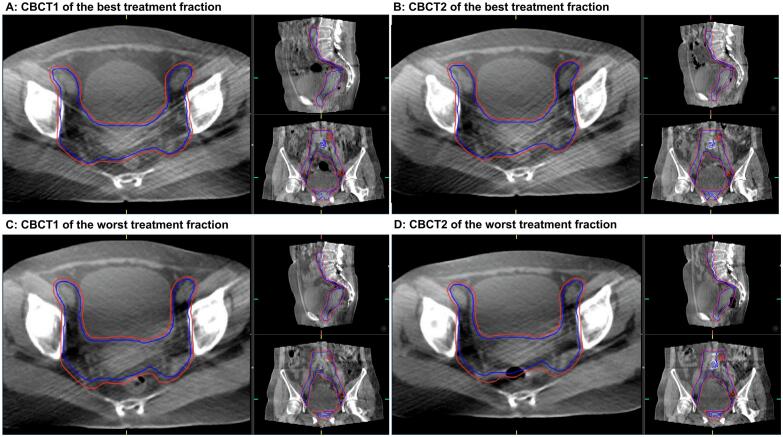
Representative images of adaptive CTV and PTV contours on CBCT pairs of one patient The best treatment fraction (A-B) and the worst fraction (C-D) of one patient were shown. The adaptive CTV(bule) and the 3-mm PTV (red) contours were superimposed after pre- and posttreatment CBCT matching with respect to bony anatomy. During the best treatment fraction (A-B), 100% coverage with minimal anatomical variations were observed on the two set of CBCTs over a 20-minute intrafraction interval. Even during the worst treatment fraction when the bowel movement on the CBCT2 (D) was rather obvious compared to that on the CBCT1 (C), still the adaptive CTV and 3-mm PTV margin ensures the target coverage on the CBCT2. (For interpretation of the references to colour in this figure legend, the reader is referred to the web version of this article.)
